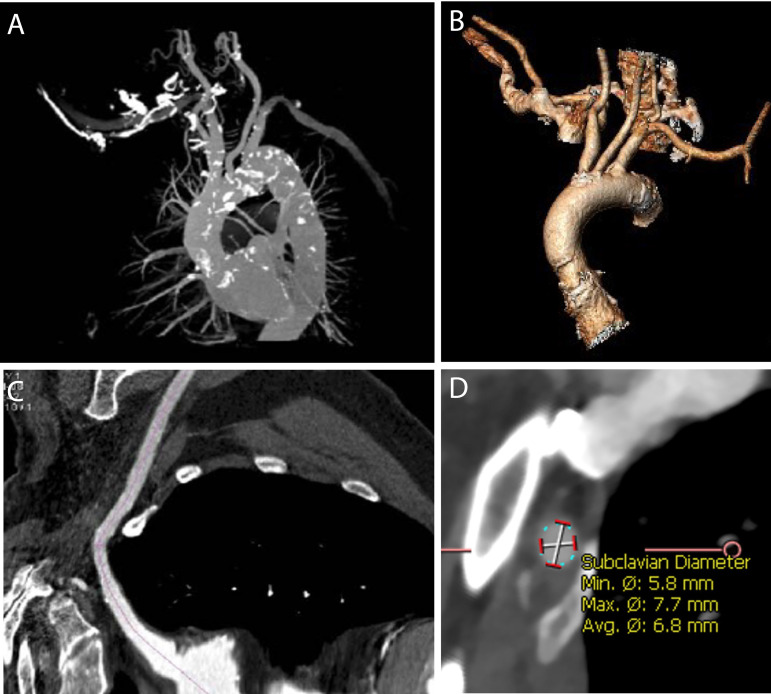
Transaxillary access evaluation. Axillary artery reconstruction in computed tomography (A-B). Vessel diameter, degree of tortuosity, relationship with side branches, and presence and extension of calcifications evaluation (B). Mean diameter suitable to transaxillary access (≥ 6 mm).
